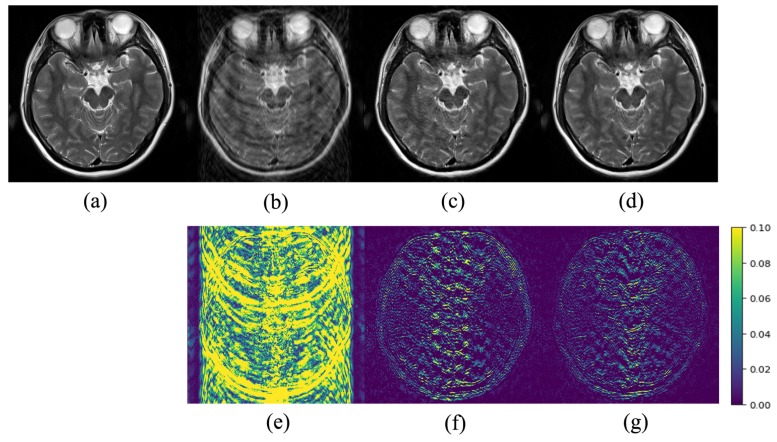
Comparison of the reconstruction results of the target MR image: (a) in Brain C using the Cartesian undersampled mask with 30% sampling rate; (b) zero-filling reconstruction; (c) DIP reconstruction; (d) the proposed method reconstruction and corresponding error maps (e)–(g).
